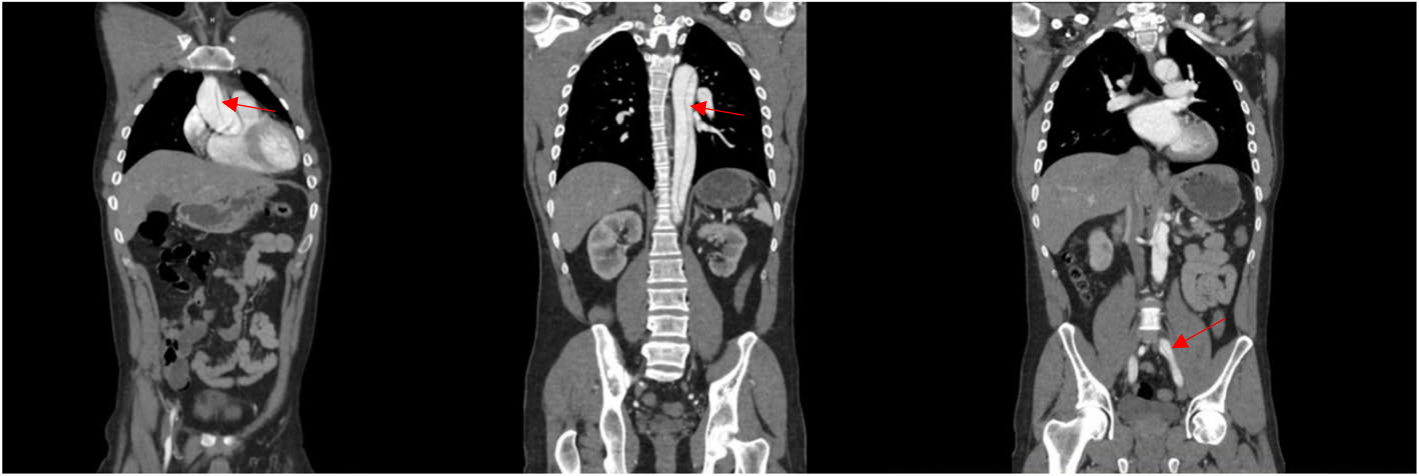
CT angiogram with contrast and coronal views depicting type A aortic dissection extending proximally to distally (left to right) to the iliac arteries and common femoral arteries bilaterally
